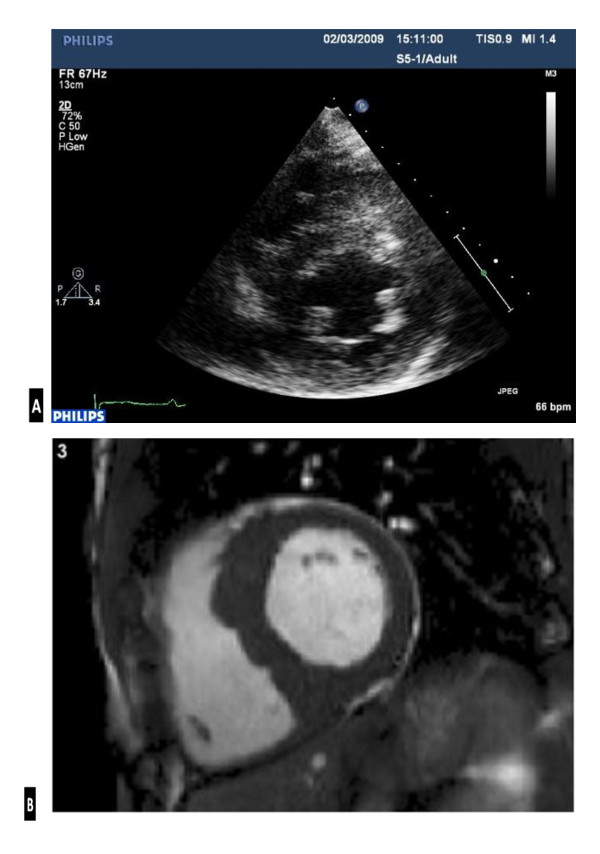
Trans-thoracic echocardiography (a) vs. CMR (b) image demonstrating; asymmetric septal hypertrophy of 14 mm and a left ventricular cavity size of 44 mm in the parasternal short axis at papillary muscle level (a) vs. asymmetric septal hypertrophy of 17 mm, a left ventricular cavity size of 44 mm and a lateral wall of 8.5 mm at the same level (b).
